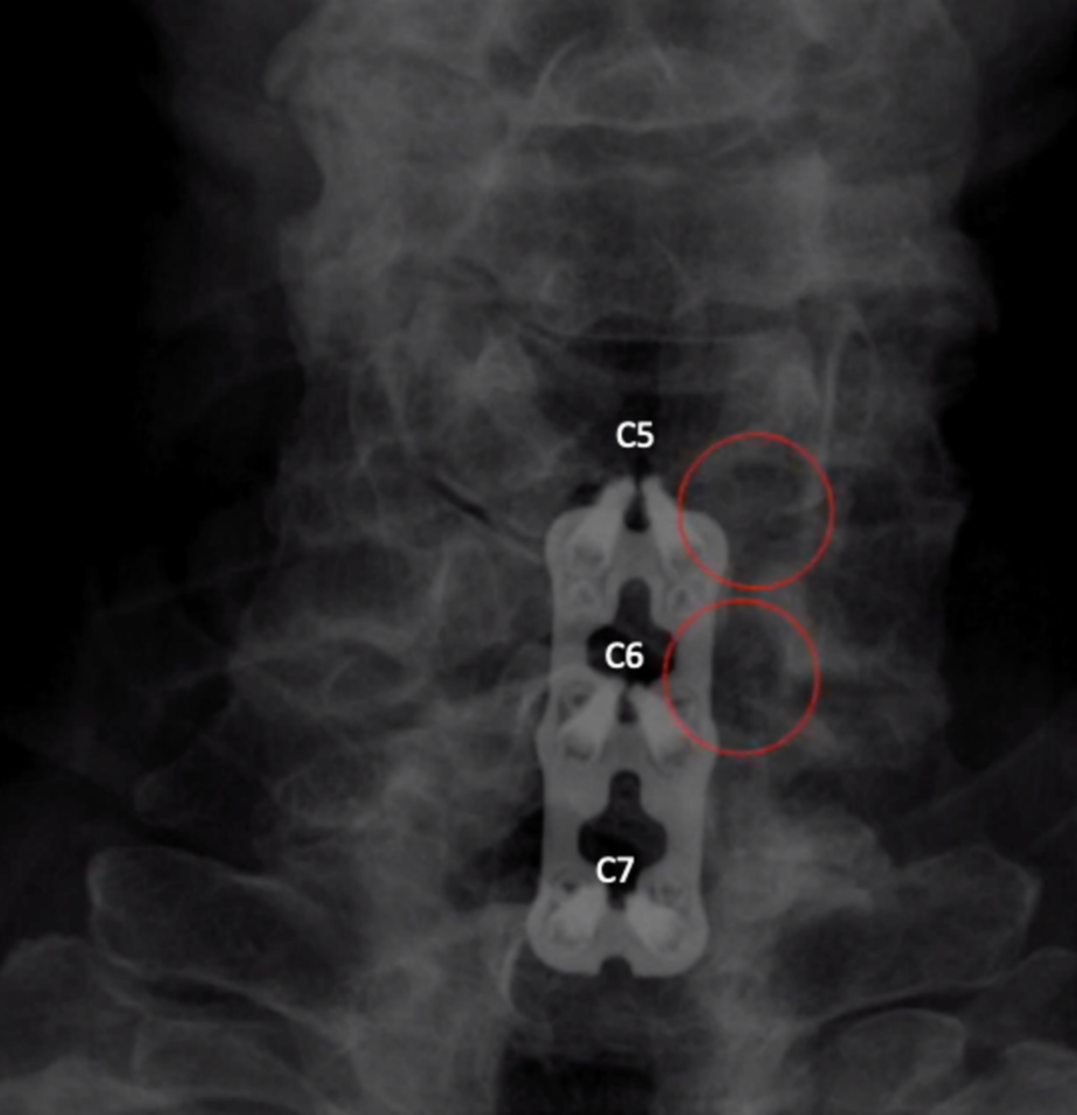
Postoperative X-ray after C5-7 ACDF with total uncinatectomies The image demonstrates good placement of hardware, with lucency in the C5-6 and C6-7 foramen (red circles) demonstrating complete removal of the uncinate and total decompression of the cervical roots ACDF: anterior discectomy and fusion
